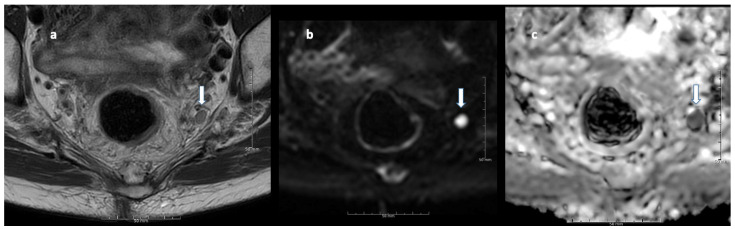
A 10 mm large extramesorectal lymph node (lateral LN) at the left internal iliac indicated with arrows at T2W image (a), diffusion weighted MRI b800 (b), and with low signal on the apparent diffusion coefficient (ADC) map indicating diffusion restriction (c).
